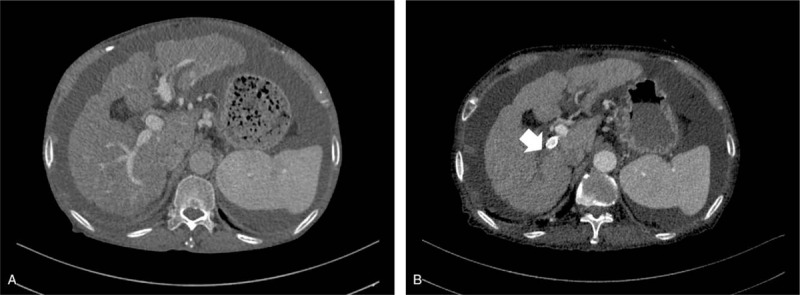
Typical de novo PVT found after TIPS using Viatorr stent in a patient by computed tomography (CT). No PVT was observed before shunt creation (A). 40 d after TIPS, thrombosis of right portal branch was confirmed by contrast-enhanced CT (B). The white arrow indicates absence of contrast in right portal branch.
